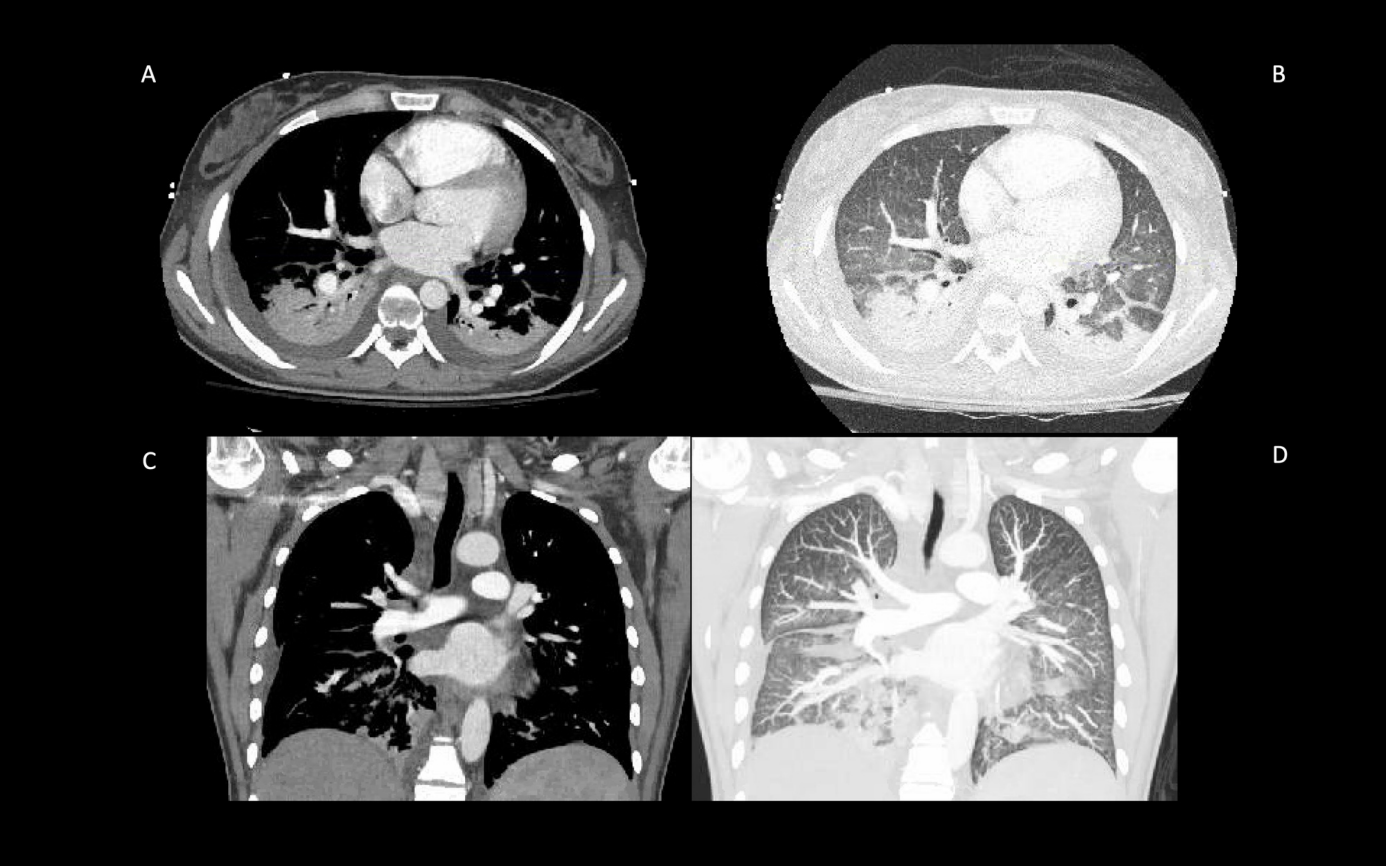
Axial CT without contrast (A), axial CT with contrast (B), coronal CT without contrast (C), and coronal CT with contrast (D) showing no abnormal findings.
